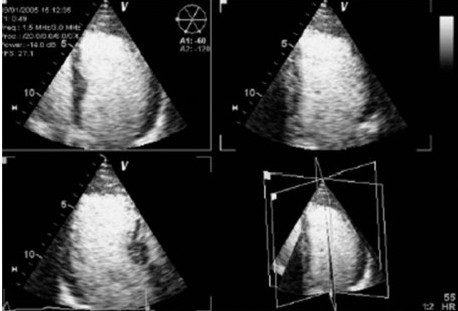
Example of contrast RT3DE triplane showing the apical three orthogonal cutting planes.
